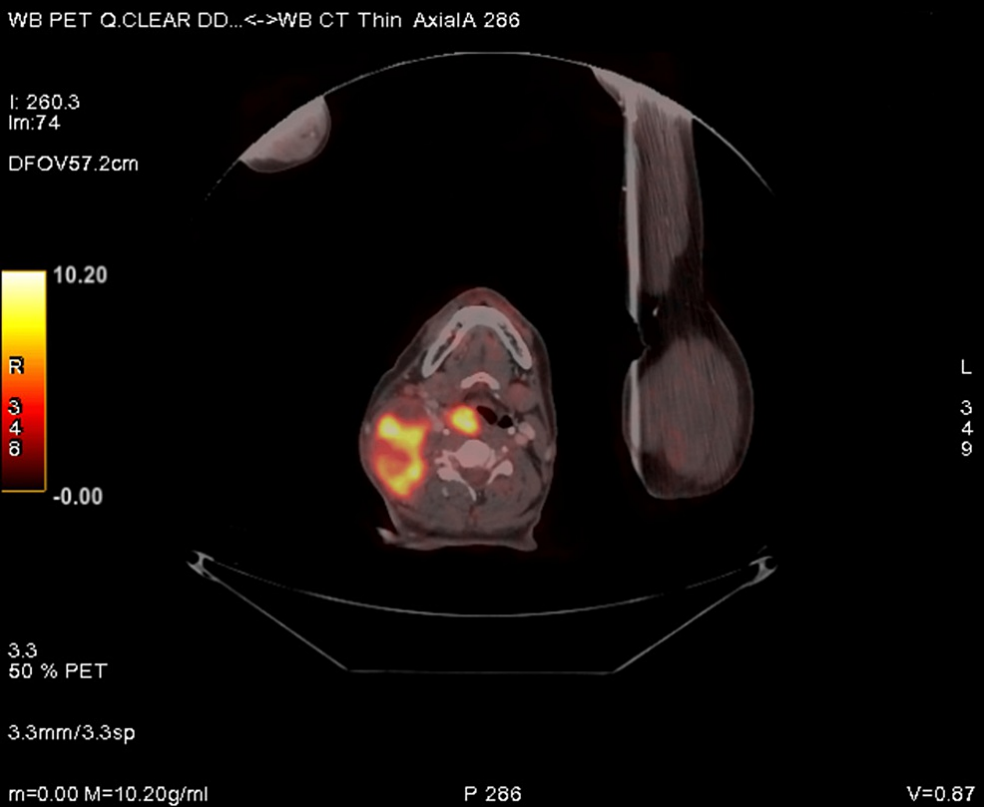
PET-CT showing FDG avid heterogeneously enhancing enlarged right cervical level II, III, IV, Vb, and right retropharyngeal lymph node.
